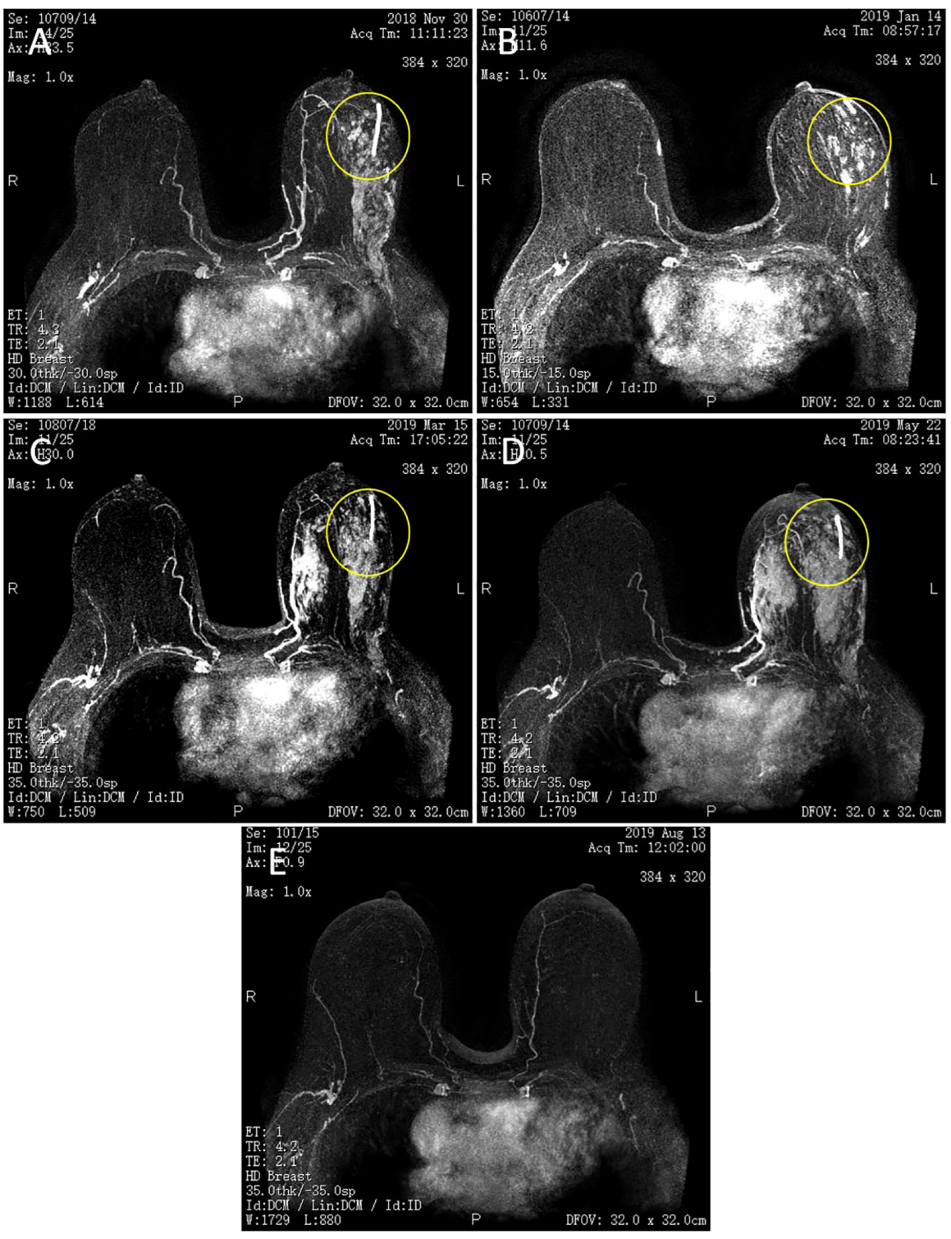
Magnetic resonance imaging (MRI) of bilateral breasts. (A) Two cycles of TEC. (B) Four cycles of TEC. (C) Two cycles of NP. (D) Four cycles of NP. (E) Capecitabine + FUL for 3 + months. The left breast lesion has disappeared. The yellow circle shows that left breast lesion.
